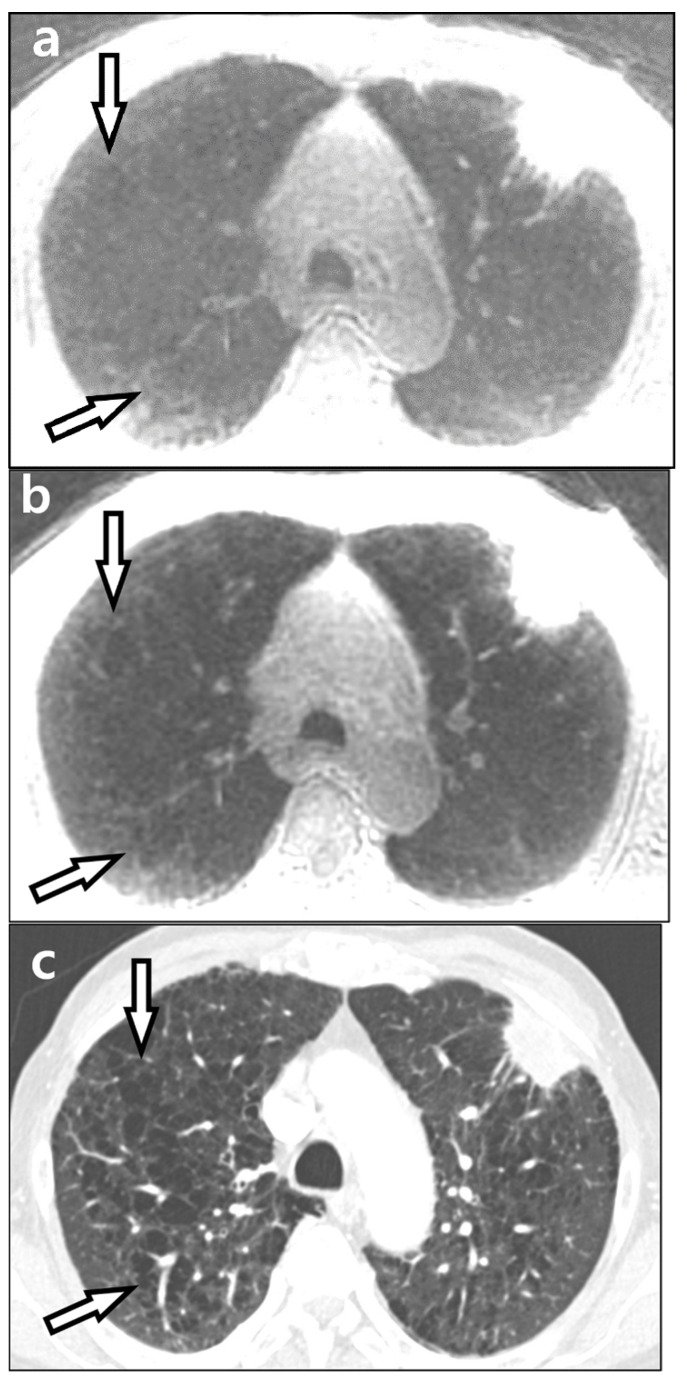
An 83-year-old male with lung cancer. A lobulated mass in the left upper lobe is well-defined in both ZTE-CAA (a) and ZTE-AIR (b). However, the presence of emphysema is more clearly defined in ZTE-AIR. CT image is presented for comparison (c). ZTE-CAA, zero echo time lung MR image using conventional anterior array coil; ZTE-AIR, zero echo time lung MR image using adaptive image receive coil.
